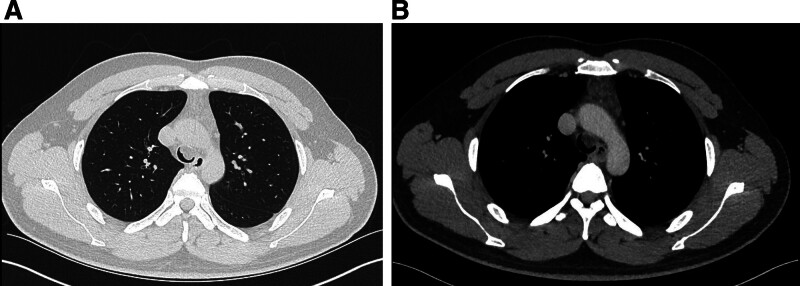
Contrast-enhanced chest CT. (A, B) Above the carina of trachea a nodular shadow is observed in the trachea adjacent to the anterior wall, protruding inward of the cavity.
